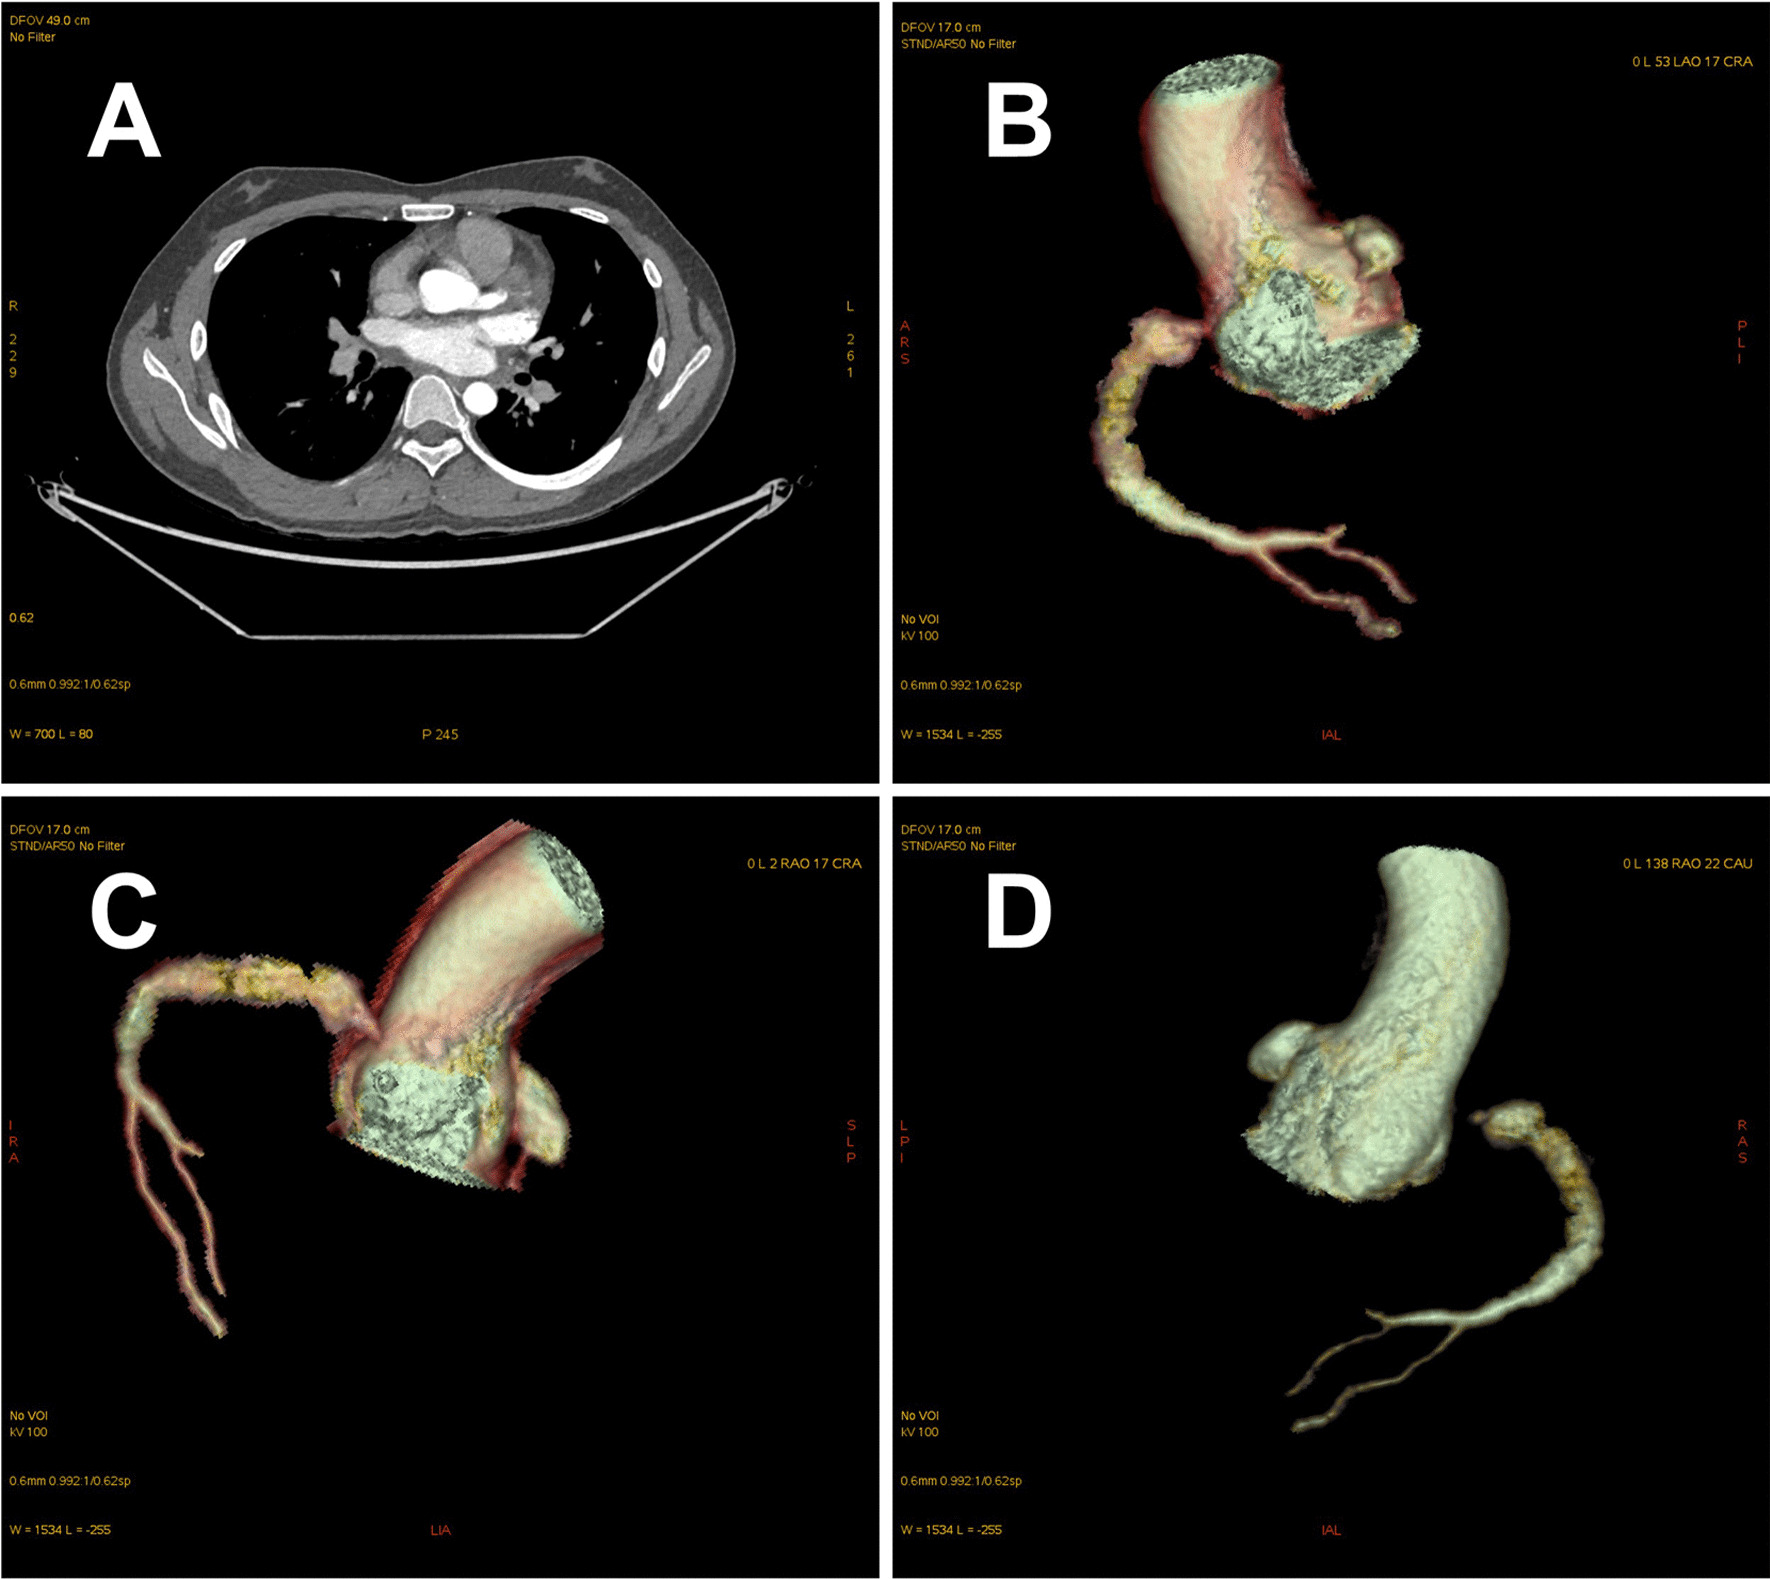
Coronary CT angiography showing the presence of aneurysms in left main coronary artery (LMCA) and right coronary artery (RCA)
